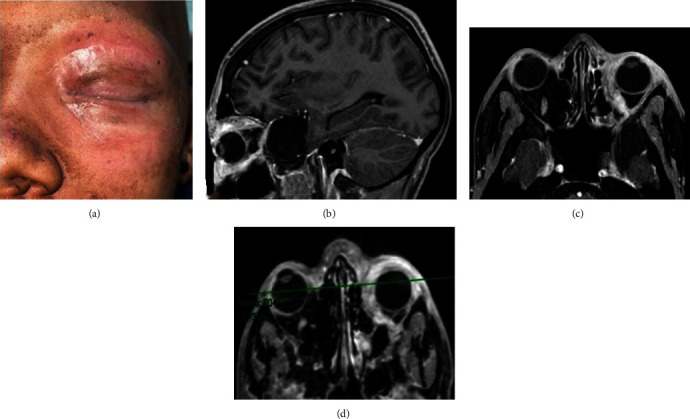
Clinical and imaging analyses after final RT session. (a) Reduced tumor volume was observed and by MRI in sagittal (b) and axial (c, d) slices that showed complete response to treatment with LCR obtained.
